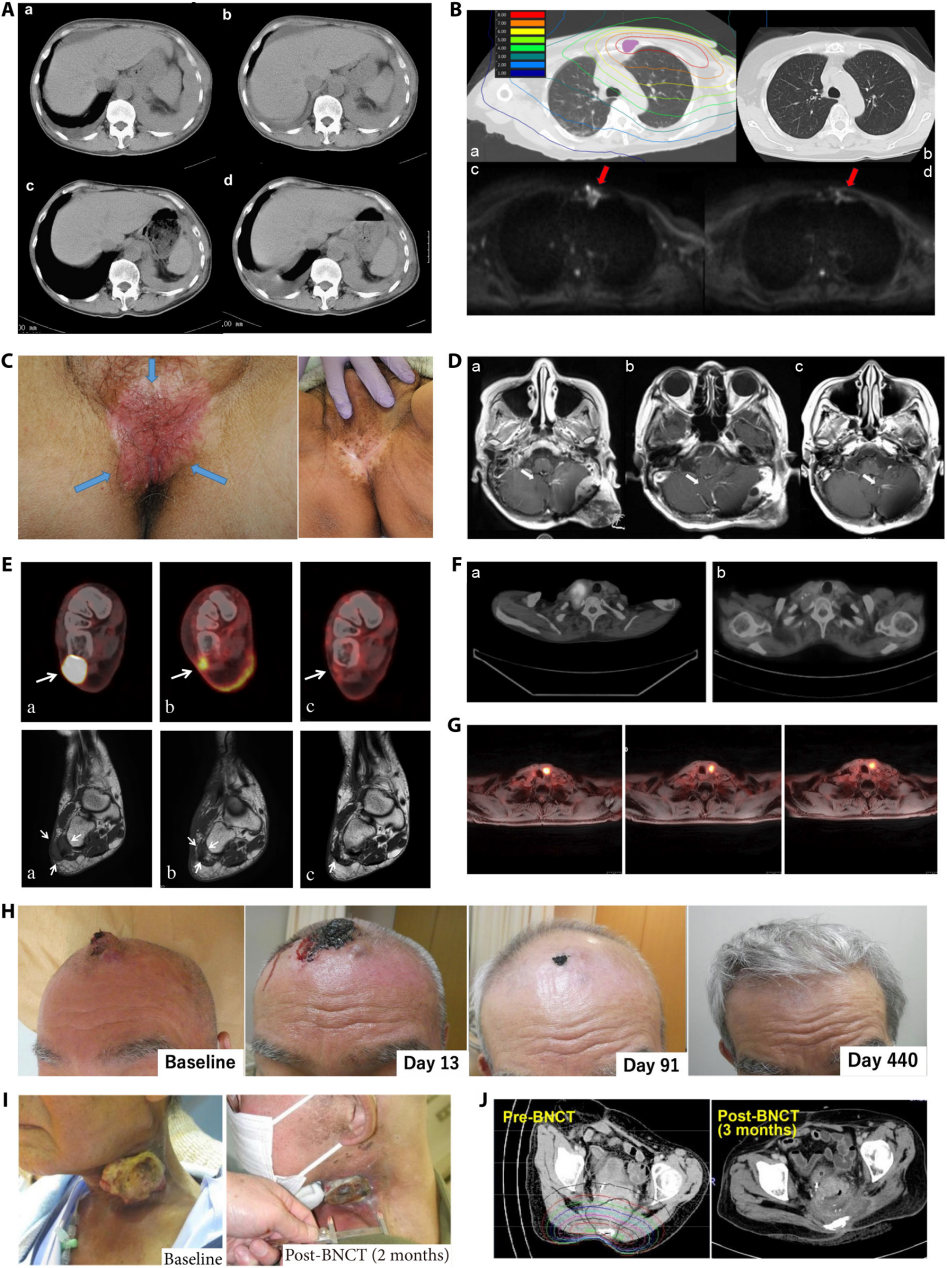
(A) CT images of a patient with malignant pleural mesothelioma are shown [170]. (a) Pre-BNCT. (b) Slight tumor regression at 1 month. (c) Partial response with tumor regression at 6 months. (d) Tumor enlargement near thoracic vertebrae at 7 months. (B) Lung dose distribution map during BNCT for recurrent breast cancer, with purple indicating gross tumor volume (a); CT scan 90 d after BNCT showed no radiation pneumonitis (b); diffusion-weighted imaging MRI revealed the tumor (red arrow) before treatment (c); MRI at 90 d after BNCT showed reduced tumor signal intensity (d) [174]. (C) Male patient with extramammary Paget’s disease [155]. Erythematous perineal lesion before BNCT (left); complete tumor regression and depigmentation after BNCT (right). (D) Gd-enhanced T1-weighted MRI of radiation-induced osteosarcoma during BNCT treatment [176]. Tumor mass visible subcutaneously and epidurally 1 month pre-BNCT (a); tumor volume reduced at 4 d after BNCT (b) and further reduced at 3 months post-BNCT (c). (E) Treatment course of clear cell sarcoma of the right foot peroneal tendon [177]. First row of pictures are ^18^F-FDG-PET/CT images. High FDG uptake (SUVmax 22.8) before first BNCT, no metastasis (a); SUVmax reduced to 3.8 after first BNCT (b); no FDG uptake after second BNCT (c). The second row of pictures are MRI T1-weighted images. Tumor visible before BNCT (a’); reduced size and signal changes 1 month after first BNCT (b’); tumor no longer visible 1 month after second BNCT (c’). (F) PET-CT of malignant peripheral nerve sheath tumor [178]. The pre-BNCT PET-CT shows high BPA uptake in tumor (a). FDG uptake normalized 18 months post-BNCT (b). (G) FDG-PET/MRI of recurrent papillary thyroid carcinoma before and after BNCT [181]. SUVmax was 17.8 pre-BNCT (left), 11.7 at 1 month after BNCT (middle), and 11.3 at 5 months post-BNCT (right). (H) Scalp angiosarcoma treatment course with BNCT [158]. (I) Pretreatment and 2-month post-treatment CT scans demonstrated growth suppression of the metastatic cervical gastric tumor [168]. (J) Pretreatment and 3-month post-treatment MR images showed stable tumor size in the patient with rectal cancer [168].
